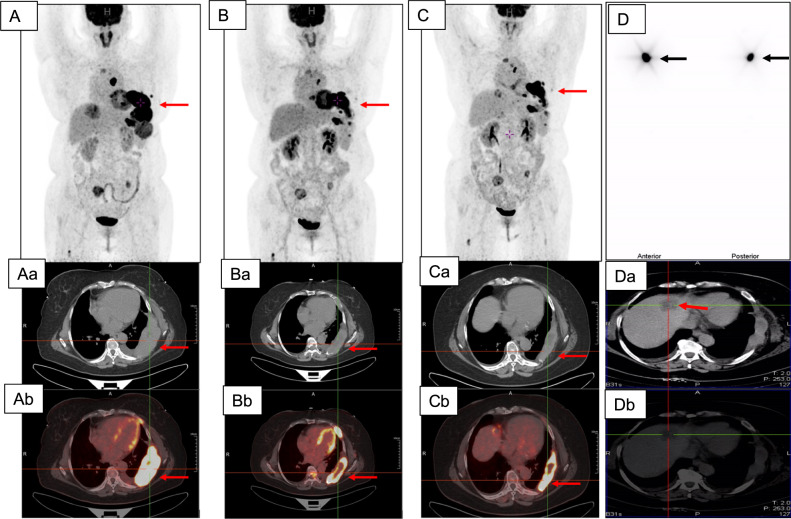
In case 4, ^18^F-FDG PET/CT revealed multiple mediastinal lymph nodes, pulmonary, liver and left 5^th^ to 9^th^ ribs as well as left thoracic wall metastases (A, Aa and Ab). Decreased tumour size and SUVmax after 4-month therapy with apatinib (B, Ba and Bb). However, no further decrease of tumour size was observed after apatinib therapy combined with RAI (C, Ca and Cb). Red arrows indicated metastases in left 5^th^ to 9^th^ ribs as well as left thoracic wall. The negative post-therapeutic ^131^I whole body scans in anterior and posterior views (D) and CT (Da) as well as SPECT/CT (Db) of liver metastasis were shown. The black arrows in D indicated thyroid remnant
